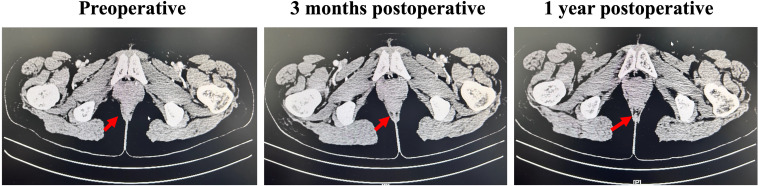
Abdominal CT images at various time points. Red arrows indicate the patient’s anal canal surgical area.
